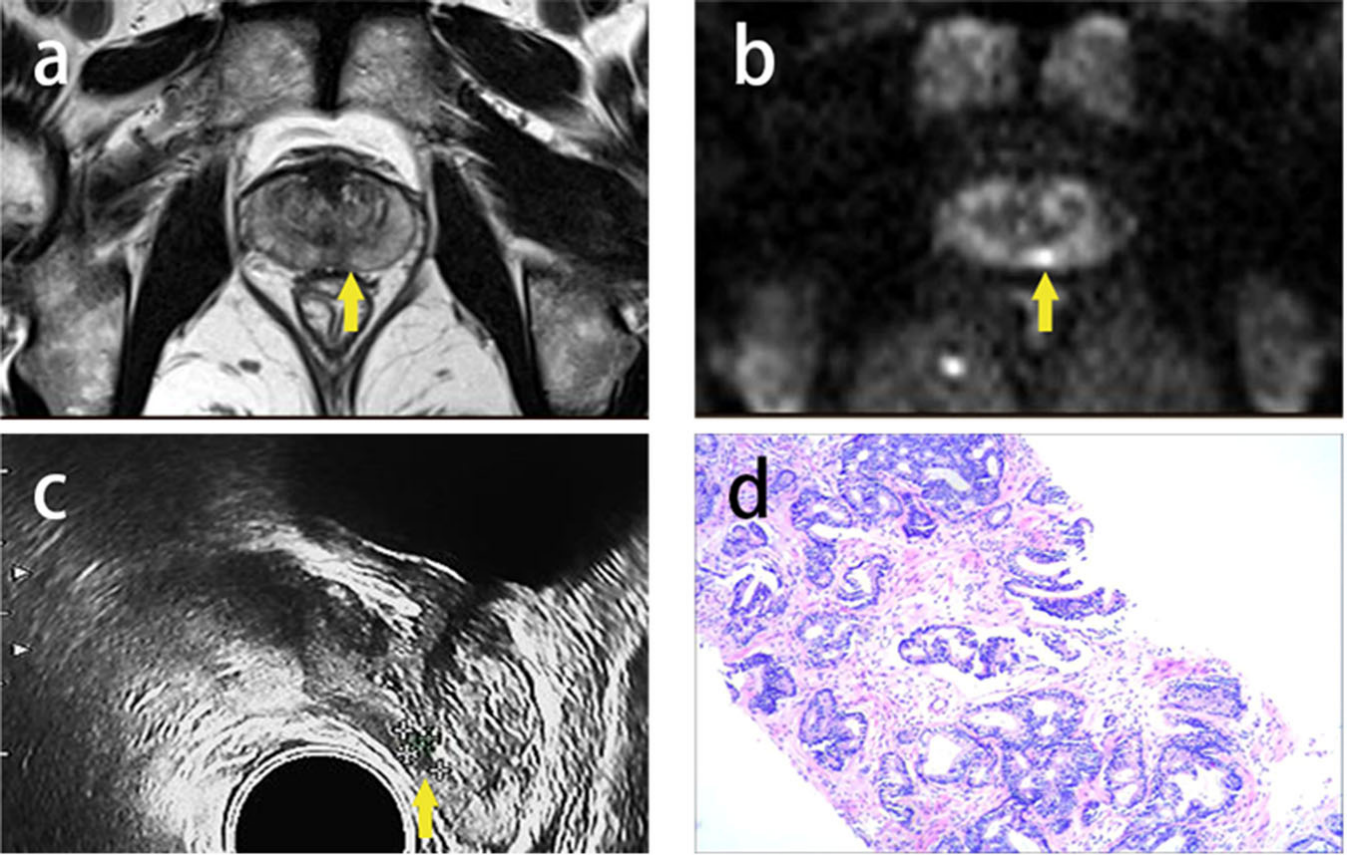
Imaging in a 67-year-old man with prostate-specific antigen 8.8 ng/mL. The suspected PI-RADS 3 lesion was in the left PZ of midgland. a Mildly hypointensity on T2WI (arrow); b Mildly hyperintense on DWI (arrow); c Hypoechoic on transrectal ultrasound (arrow); d Prostate adenocarcinoma, Gleason Score 4 + 3 (SUM = 7). T2WI, T2-weighted imaging; DWI, diffusion-weighted imaging
